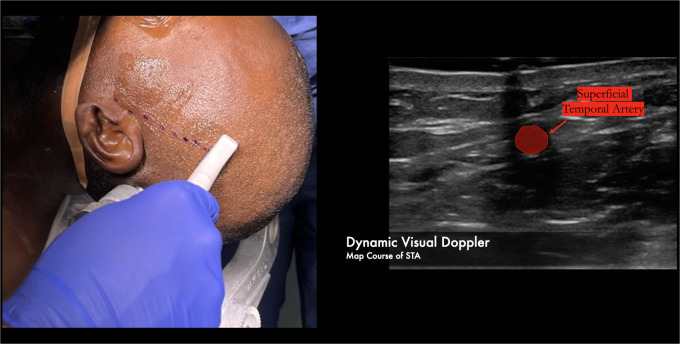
Over 10cm of the parietal branch can be routinely harvested by using a combination of the visual and audio dopplers to map the course of the donor vessel.
